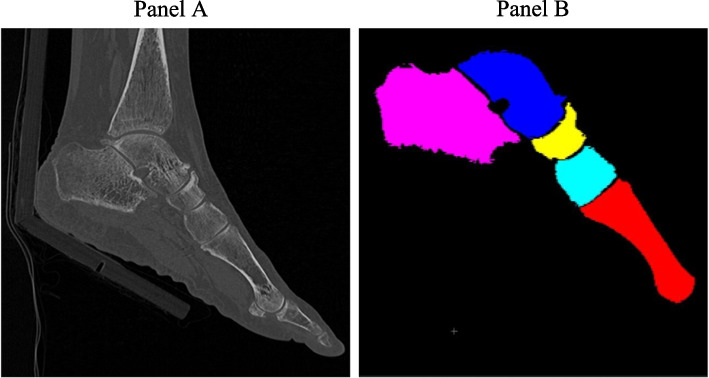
Images of Foot Positioning for CT scan and Segmentation of foot bones. Panel A CT scan of target leg resting on a stabilization board holding the ankle in 30 degrees of plantar flexion and metatarsophalangeal joints in the resting position; Panel B Sagittal cross-section of segmentation of tarsals and metatarsals (from left: calcaneus, talus, navicular, 1^st^ cuneiform, 1^st^ metatarsal)
